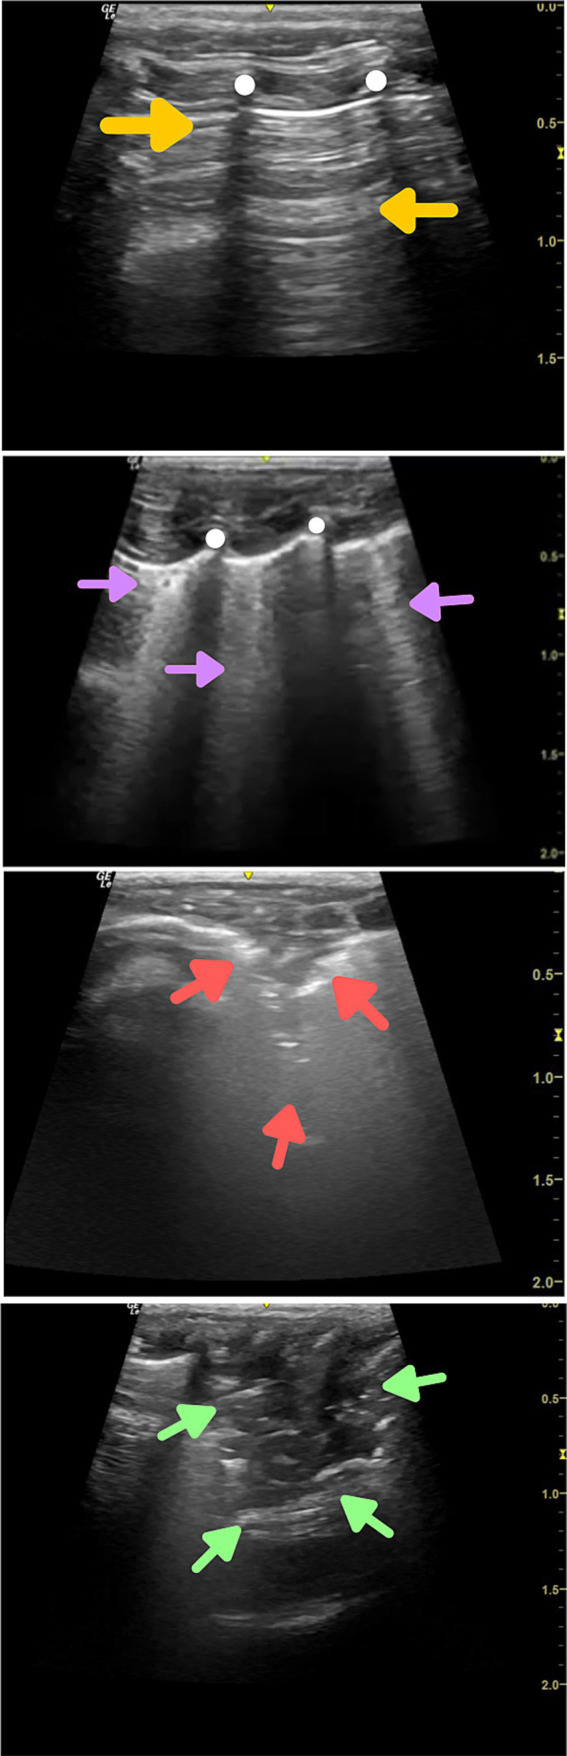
Thoracic ultrasound findings from *Callithrix* sp. (A) Healthy lung parenchyma, with the presence of parallel horizontal lines starting in the subpleural region and extending to the distal field (lines A—profile A) (yellow arrowheads). (B) Presence of parallel vertical hyperechoic artefacts starting in the subpleural region and extending to the distal field (lines B—profile B) (purple arrowheads). (C) Presence of consolidation with air bronchograms, represented by a hypoechoic area in the subpleural region, with poorly delimited outlines and the presence of vertical hyperechoic lines within the area described (profile C) (red arrowheads). (D) Presence of consolidation with static bronchogram, represented by a triangular-shaped hypoechogenic area, without vertical hyperechogenic lines inside the area described (green arrowheads).
